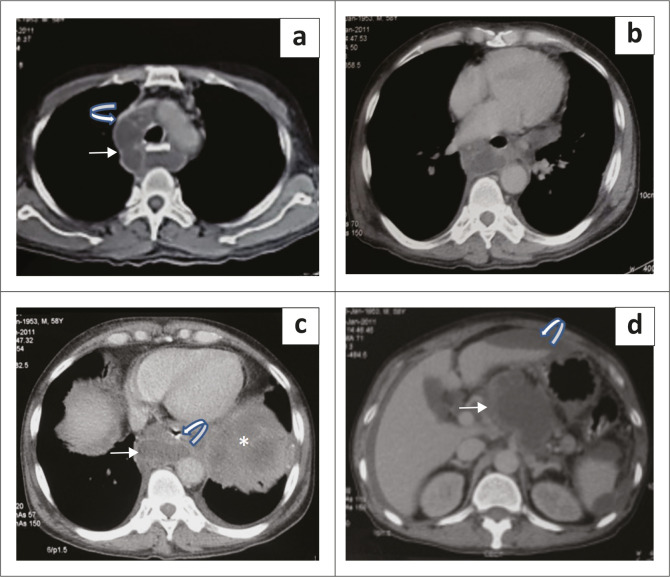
Axial sections of contrast-enhanced CT scan of the chest and abdomen (a–d) show the pseudocyst in the visceral compartment of the mediastinum abutting the superior vena cava (curved arrow in a) and descending thoracic aorta, with anterior displacement of the oesophagus (curved arrow in c). It is extending into the abdomen through the oesophageal hiatus (white arrow in c) where it is seen arising from the body of pancreas (white arrow in d). Ascites (curved arrow in d), bilateral pleural effusions and left lower lobe consolidation (asterisk in c) are also noted.
